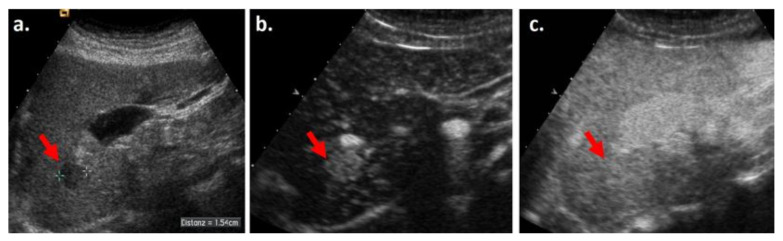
Grayscale ultrasound of the liver showing a slightly hypoechoic nodule in segment six of the liver in a cirrhotic patient (a). On CEUS, the nodule was hypervascular in the arterial phase (b) with no washout in the late phase (c), and it was consequently classified as LI-RADS 4. On CT, the nodule was isoenhancing to the liver parenchyma in all phases of enhancement. The nodule was a biopsy proven HCC.
